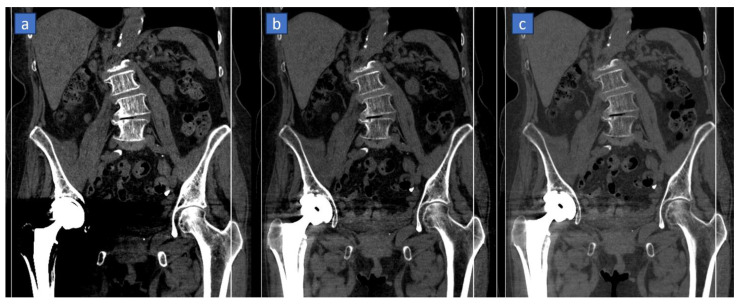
Reduction of metal-induced artifact in the pelvis of a 74-year-old woman suffering from rectal cancer. On the standard coronal CT reconstructed image, (a) heavy artifacts can be recognized around the total right hip prosthesis. The artifacts are well controlled on the monoenergetic reconstructed images obtained from DECT at 155 KeV (b) and above 170 KeV values (c), allowing for the correct visualization of pelvic structures.
